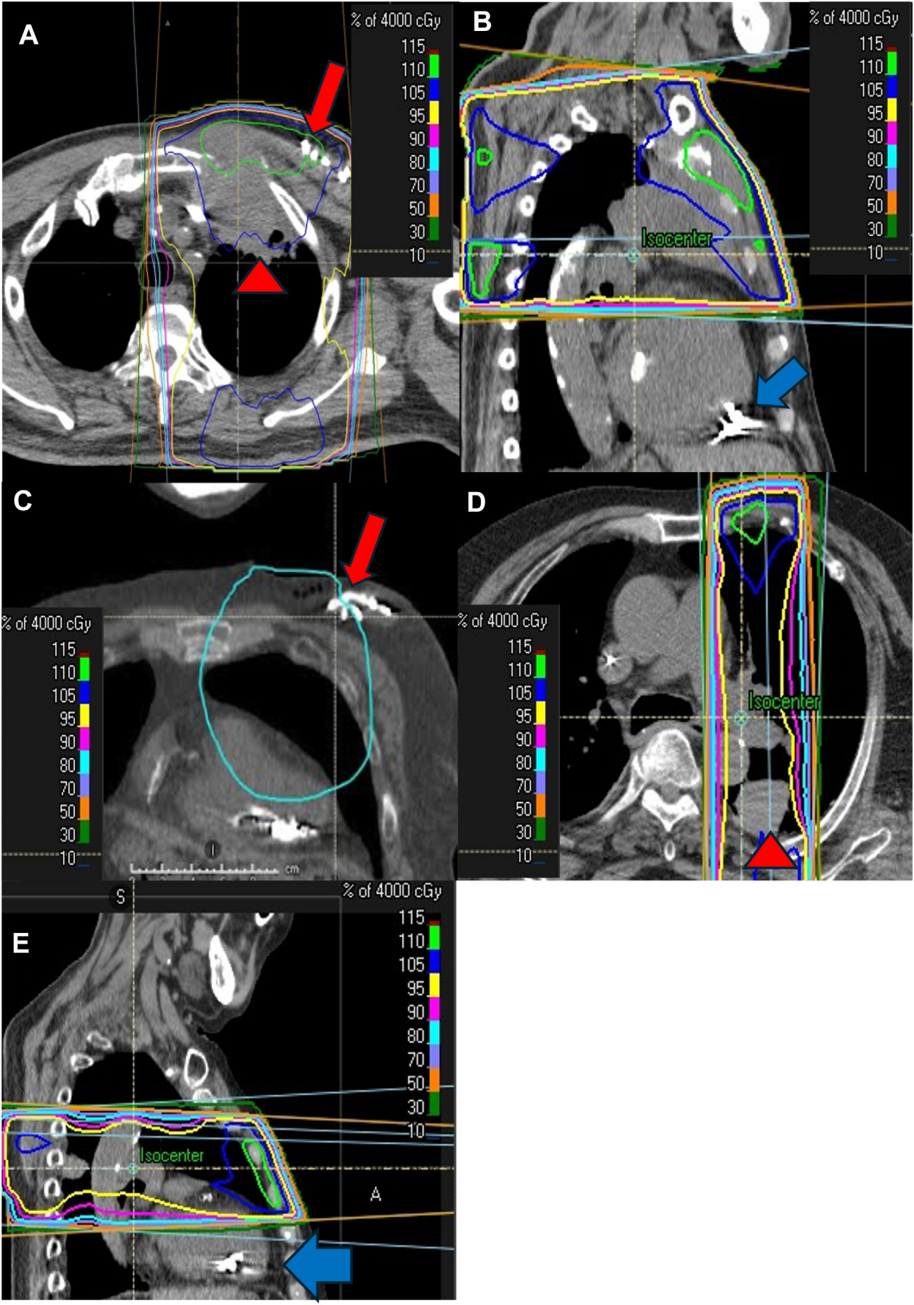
Radiotherapy planning images showing isodose lines in the axial, sagittal, and coronal planes. A, B: Images from the first case. A: Axial CT image demonstrating the lung tumor and the previously implanted pacemaker. The low-density area in the left upper lung (red arrowhead) indicates a squamous cell carcinoma requiring radiation therapy. The radiation field encompasses the pacemaker generator (red arrow), indicating a direct radiation exposure of approximately 40 Gy. B: Sagittal CT image showing the Aveir VR implanted on the right ventricular apical septum (blue arrow), positioned outside the radiation field to avoid direct exposure. C, D, E: Images from the second case. C: Coronal CT image acquired during expiration shows that the radiation field includes the pacemaker generator (red arrow), indicating a direct radiation exposure of approximately 30 Gy. D: Axial CT image showing a large-cell neuroendocrine carcinoma in the left lower lobe (red arrowhead). E: Sagittal CT image showing the Aveir VR located in the right ventricular apical septum (blue arrow), placed to avoid direct radiation exposure. CT = computed tomography.
